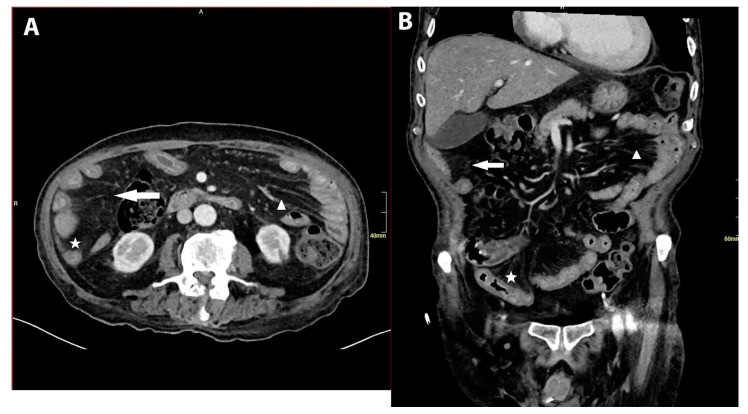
(A,B): Abdominal CT with signs of infectious enteritis. Axial (A) and coronal (B) contrast-enhanced CT images showed signs of an infectious enteritis. The capture shows diffused small bowel wall thickening with mucosal hyperenhancement (asterisk) and perienteric fat stranding (arrow). It also shows mesenteric vessel engorgement (arrowhead). The small bowel was affected entirely from the duodenum to the ileocecal valve. We did not confirm ischemic colitis. Conclusion: CMV infection is usually considered in patients with AIDS, hematological malignancies or after tissue and organ transplantation; however, tissue-invasive CMV disease can also appear in an immunocompromised patient due to long-term corticosteroid therapy.
